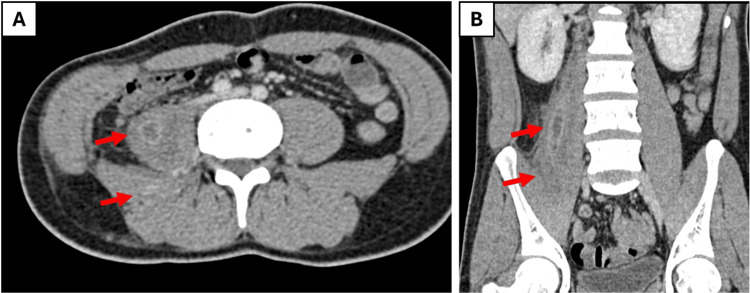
Follow-up (two-week interval) selected axial (A) and coronal (B) contrast-enhanced CT images demonstrate significant regression of the previously noted multiloculated abscess within the right psoas and iliacus muscles The collection has markedly decreased in size, with resolution of muscle swelling and surrounding inflammatory changes.
